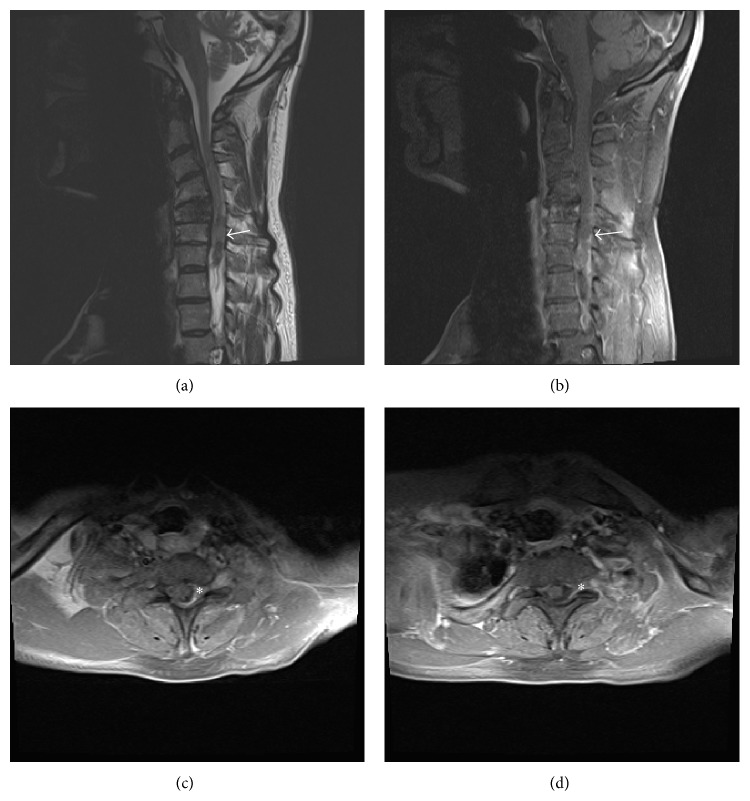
Cervical MRI: (a) midsagittal T2 MRI (TR = 4000, TE = 98) and (b) midsagittal T1 postcontrast (TR = 659, TE = 8.7) imaging of the cervical spine showing a C2–C7 ill-defined extradural mass that is hypointense on T2 and enhances with contrast most predominant at C6-C7. ((c) and (d)) Two axial slices of a preoperative T1 postgadolinium contrast (TR = 591, TE = 7.3) MRI of the cervical spine passing through the C6-C7 level demonstrates an extradural, enhancing mass invading the spinal canal eccentric to the left.
